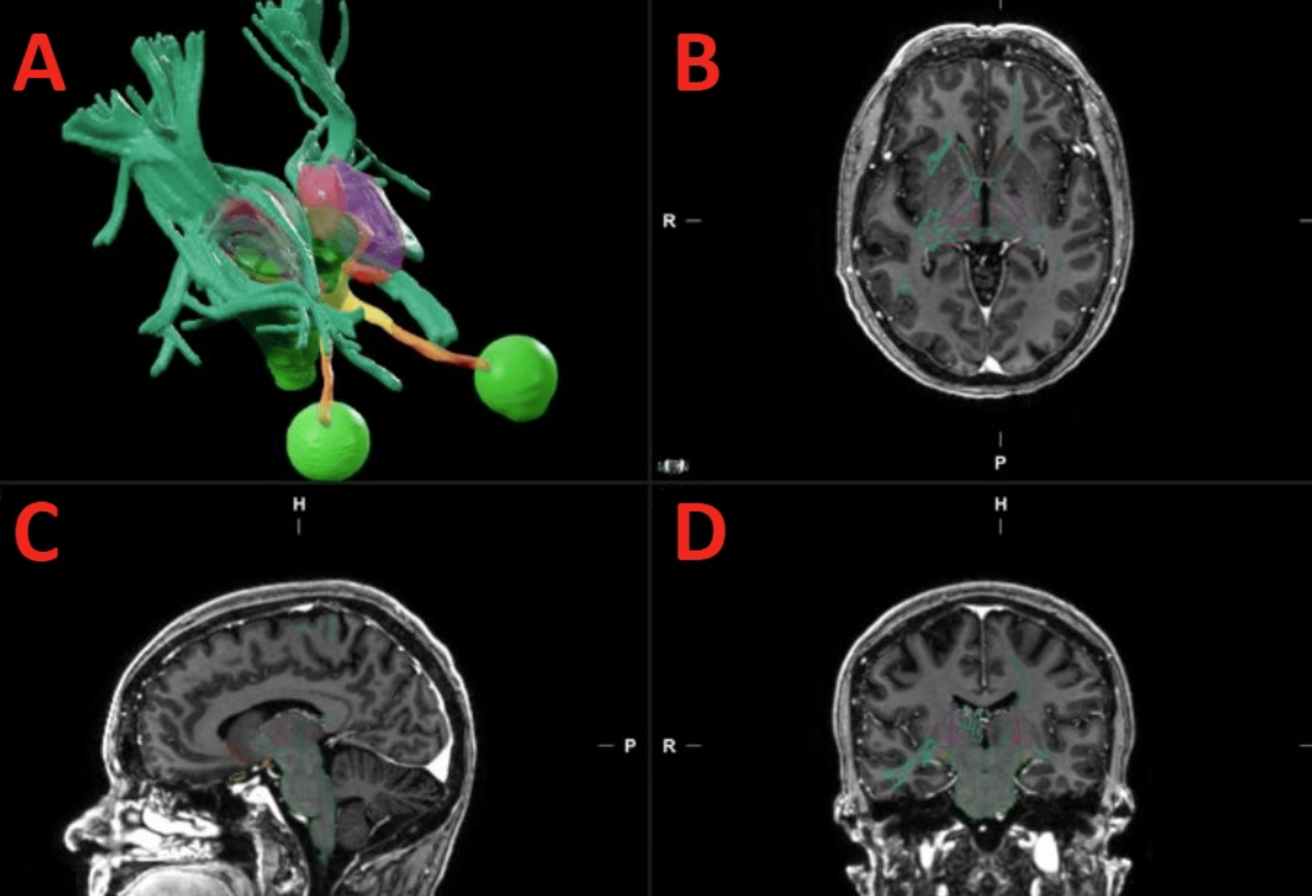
Additional critical structures Volumetric representative of the internal capsule (purple); thalamus (pink); and autosegmented radiations to and from the NAc based on DTI (teal) (A). Volumes displayed on test patient MRI in axial (B), sagittal (C), and coronal (D) views.
